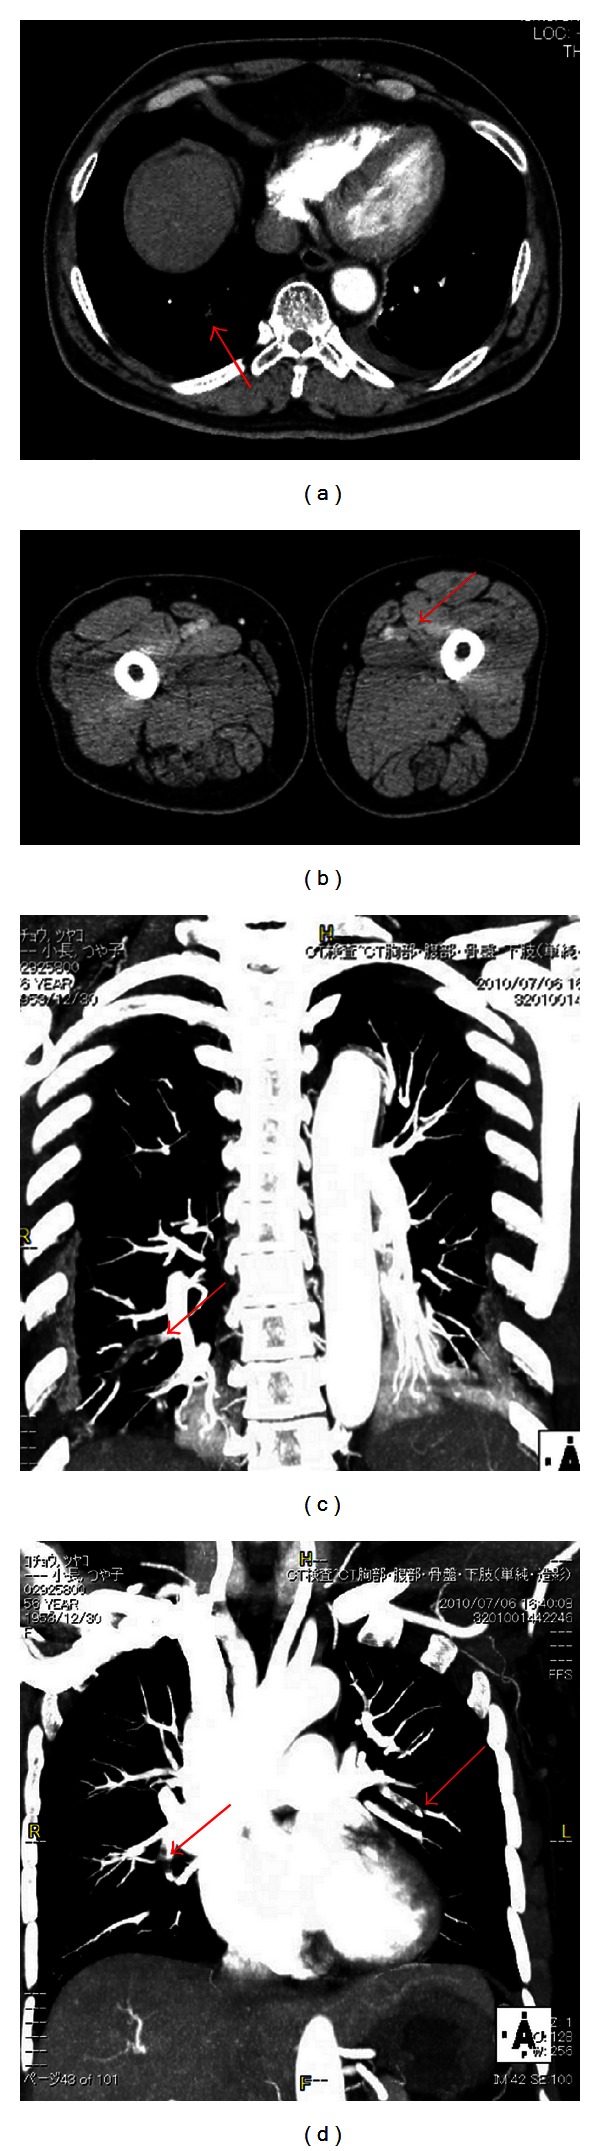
(a) Contrast-enhanced computed tomography revealed the presence of pulmonary embolism (arrow). (b) Contrast-enhanced computed tomography revealed the presence of deep venous thrombosis in left femoral vein (arrow). (c), (d). Contrast-enhanced computed tomography revealed the presence of multiple pulmonary embolisms (arrows).
